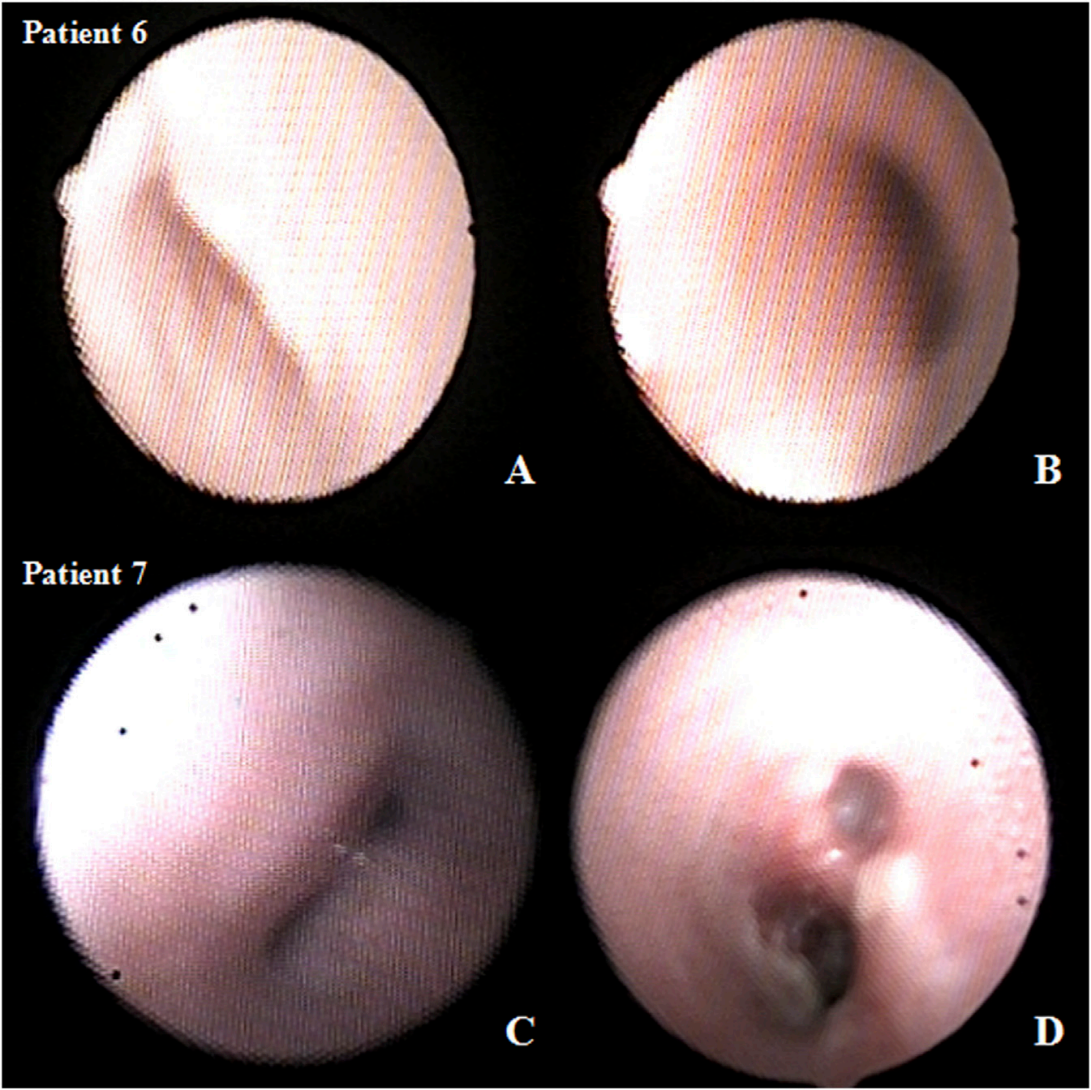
Preoperative and postoperative three-dimensional computed tomography (3D-CT) images after airway external splint suspension. (A) preoperative and (B) 1-year after operation 3D-CT images in patient six and (C) preoperative and (D) 1-year after operation 3D-CT images in patient 7.
